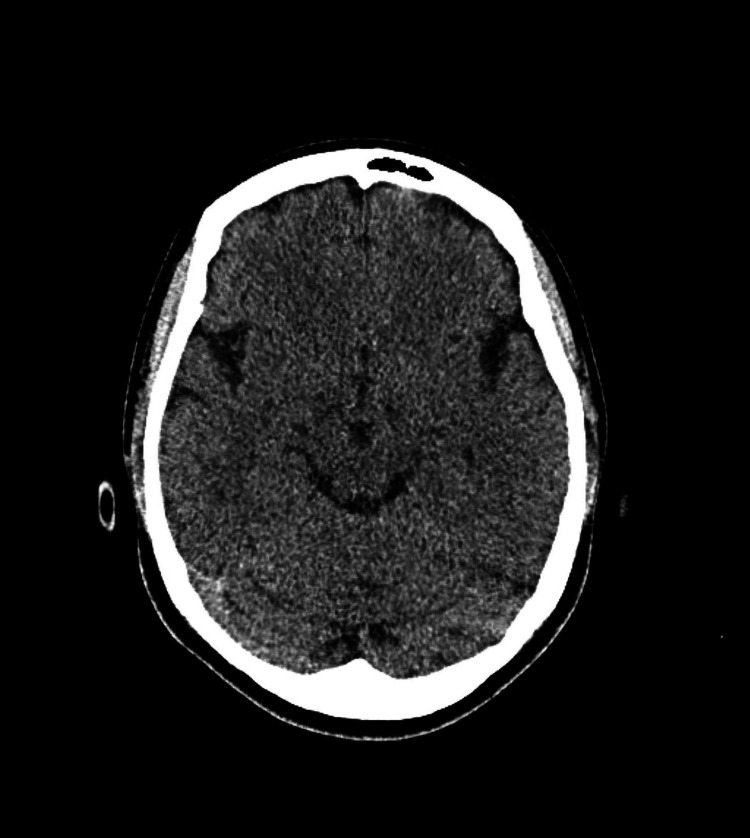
CT scan of the head showing no obvious abnormalities in the head
